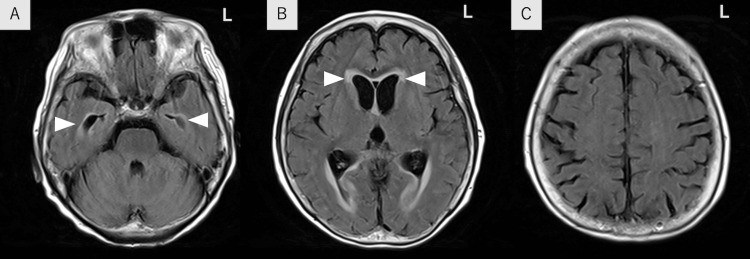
Brain magnetic resonance imaging findings on admission A-C: Brain magnetic resonance imaging showed slight hydrocephalus with periventricular hyperintensity (arrowheads). However, there were no brain lesions in fluid-attenuated inversion recovery.
